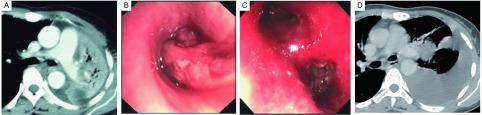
左下肺肉瘤样癌致左全肺不张。A：胸部CT显示左全肺不张，未见明确肿块；B：支气管镜显示左主支气管下端肿瘤将管腔完全堵塞；C：支气管镜下将肿瘤取出后，可见肿瘤来源于左下叶外后基底段，其他管口均畅通；D：胸部CT显示经支气管镜治疗后，左上肺复张，左肺门肿块伴胸腔积液。 Left lower atelectasis in patient with sarcoma. A: Left whole atelectasis without tumor showed in chest CT scan; B: Bronchoscopy found that left main bronchus was comletely obstructed by a tumor; C: The tumor origined from left outer basal segment when the tumor was removed by bronchoscope; D: Chest CT scan discovered that left lung reopened accompanied by left hilar tumor and pleural effusion after bronchoscopy.
